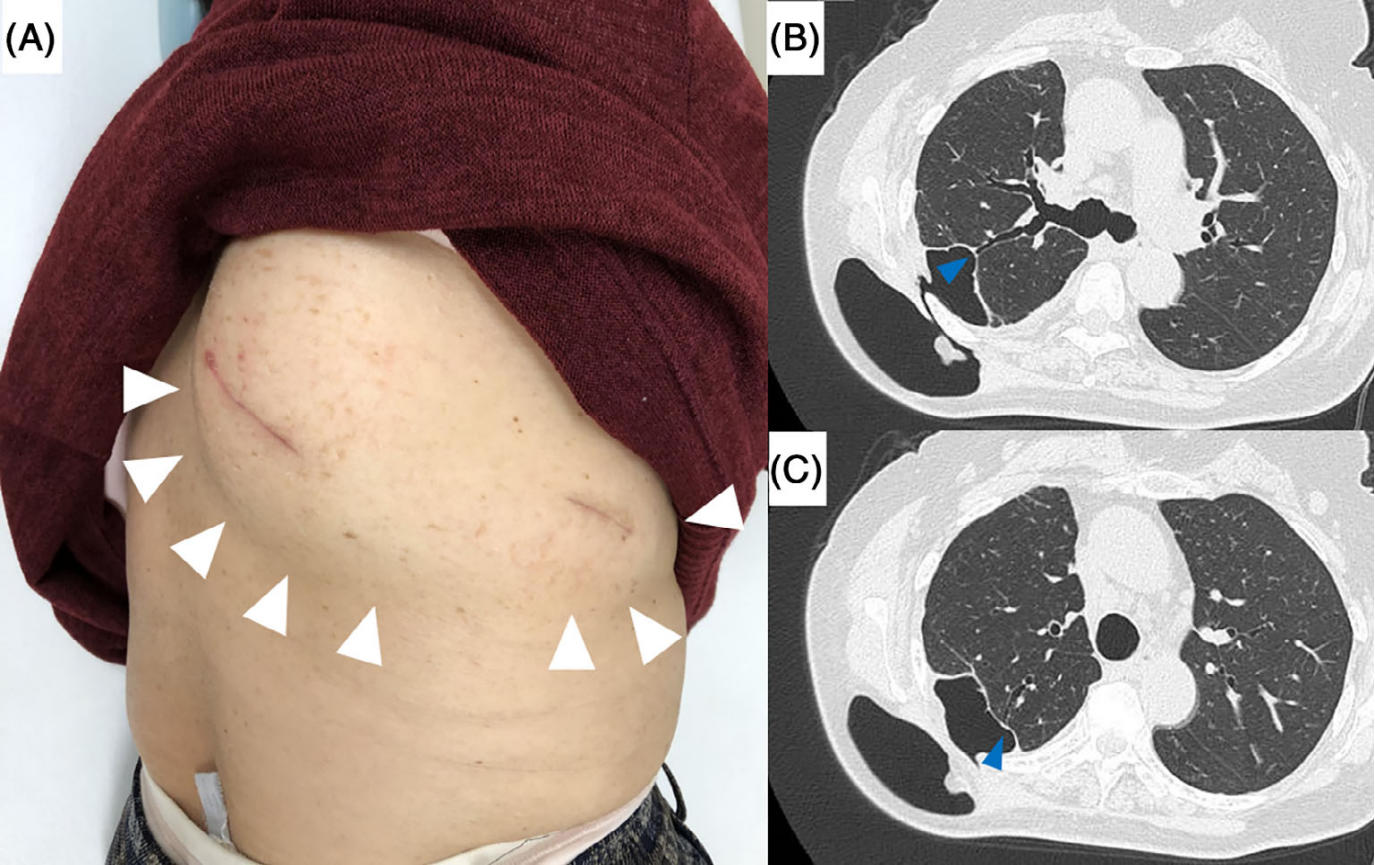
(A) Massive subcutaneous emphysema (SE) along the incisions of the right chest (white arrows). (B, C) Computed tomography of the chest showed SE and abnormal communications between subsegmental bronchi (blue arrows; (B) RB2b; (C) RB2a), pleural space, and subcutaneous tissue.
